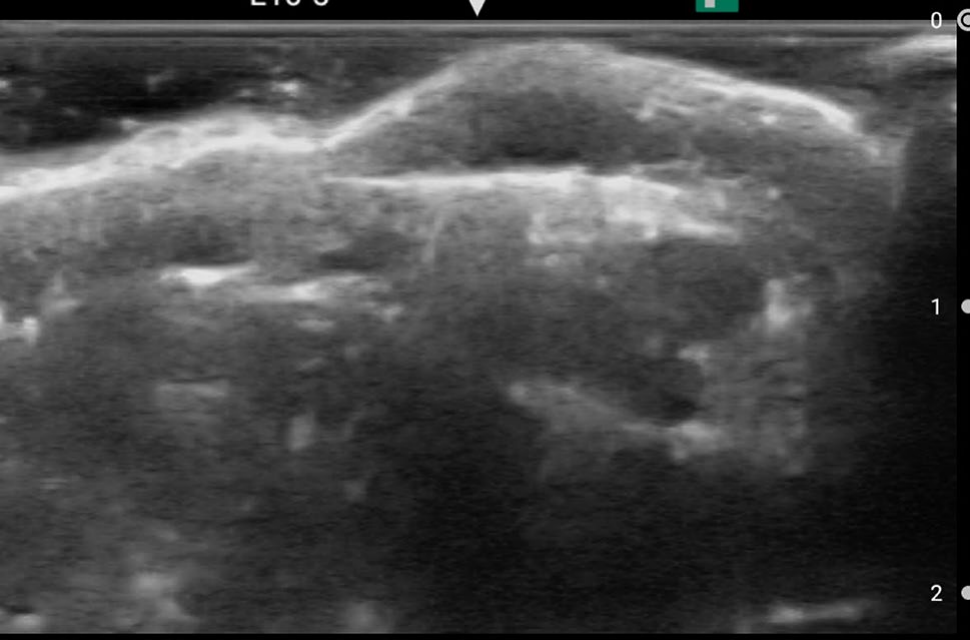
An ultrasound image which was evaluated as non-puncturable by a clinical nurse. The image was taken at right median cubital vein with the film
